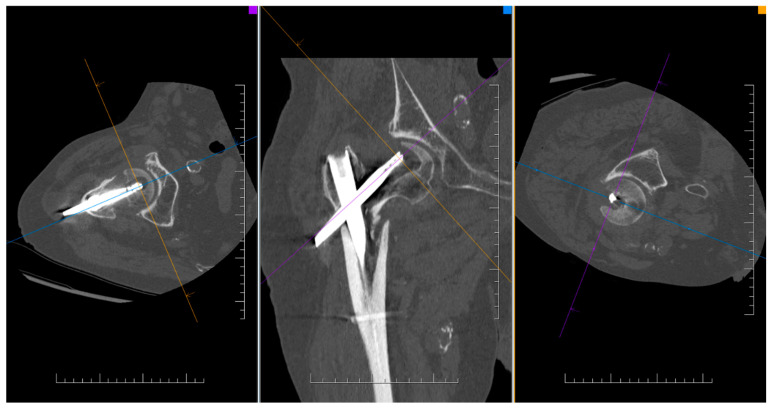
CT finding of osteosynthesis failure using TFNA without cement augmentation of the blade and with cut-out.
